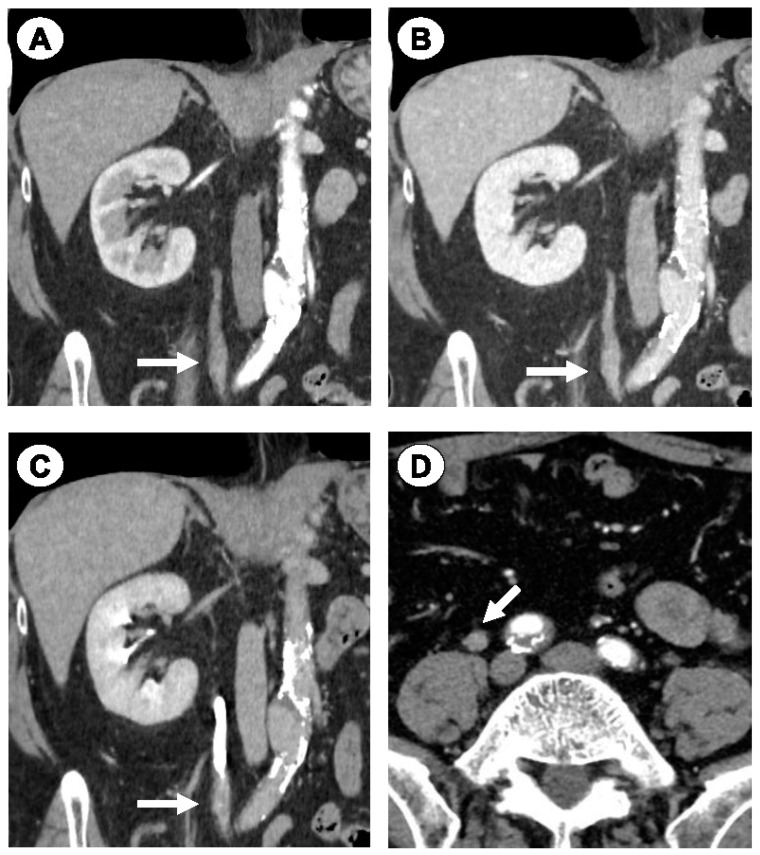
Case 3. Dynamic CT images demonstrating a right ureteral tumor. Coronal view of renal cortex enhancement (A), and parenchymal (B) and excretion phases (C). Axial view (D). Arrows indicate the tumor.
